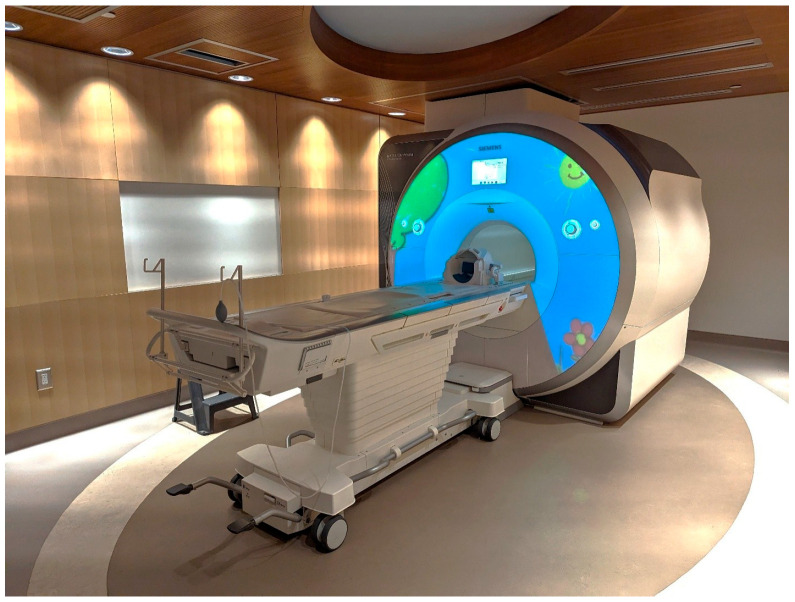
MRI suite at Holland Bloorview Kids Rehabilitation Hospital. This suite is fully accessible, child-friendly, research-focused, immersive, and customizable. Child-friendly elements of the MRI suite include child-friendly projections on the magnet bore, removable MRI scanning bed to accommodate transfers and acclimation prior to enter the scan room, customizable wall and ceiling projections, dimmable and color adjustable comfort lighting, smart glass door, and MRI-compatible audiovisual goggles (not shown). Non-sedate structural MRI of the brain was performed pre- and post-intervention, with the option of a third follow-up MRI.
